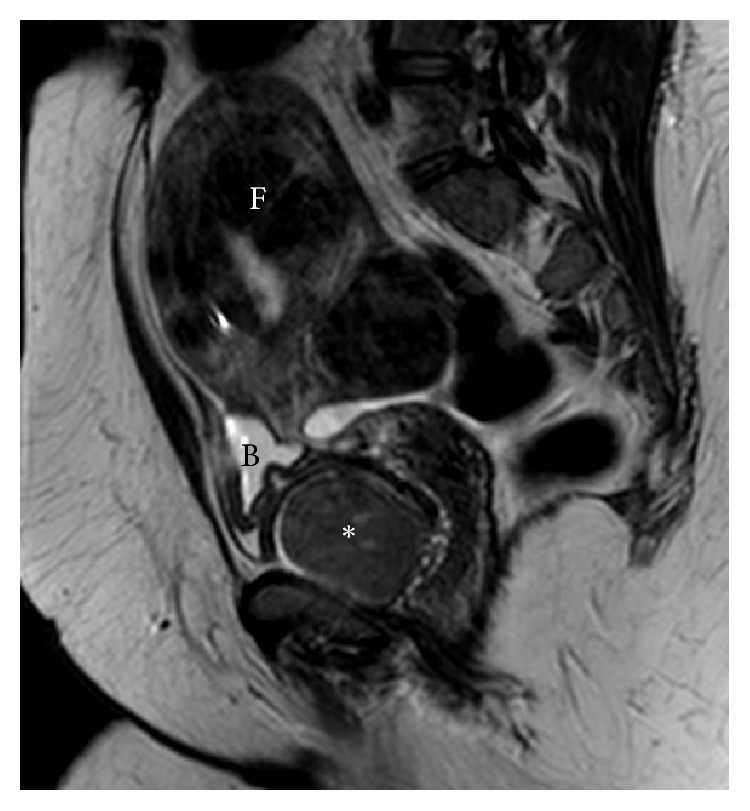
Preoperative imaging: T2-weighted pelvic MRI. F: fibroid uterus. B: bladder. ^*∗*^Paraurethral leiomyoma.
